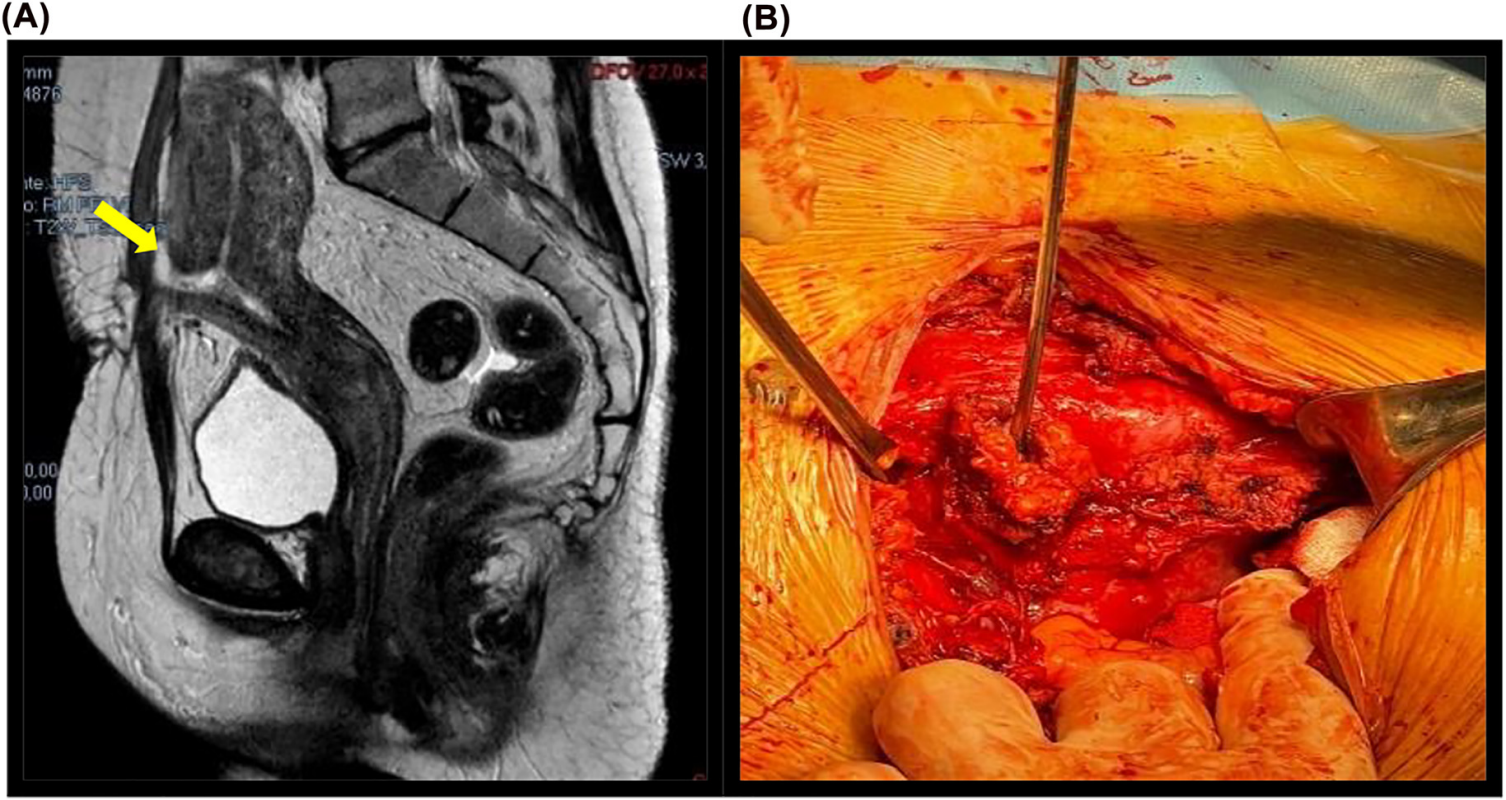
Case report 1. (A) Sagittal MRI scan of the patient showing the fistolous tract (yellow arrow) between uterine cavity and anterior abdominal wall. (B) Surgery: fistulous tract opening into the anterior wall of the uterine cavity.
